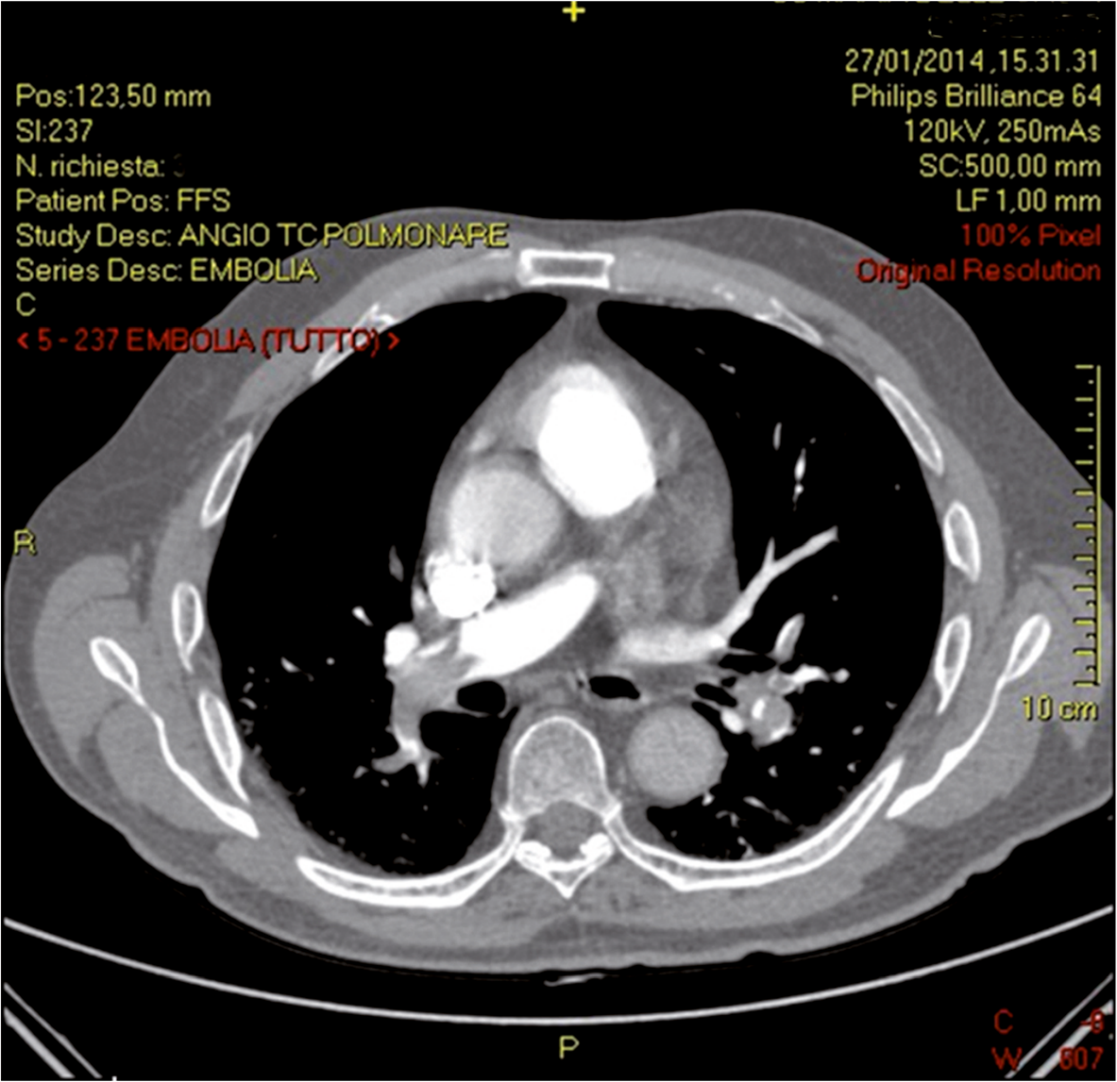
Computed tomographic pulmonary angiography on admission: multiple filling defects within the pulmonary trunk and the right pulmonary artery. Left pulmonary artery presents similar features. These computed tomography findings are suggestive of pulmonary thromboembolism (Miller index >17)
